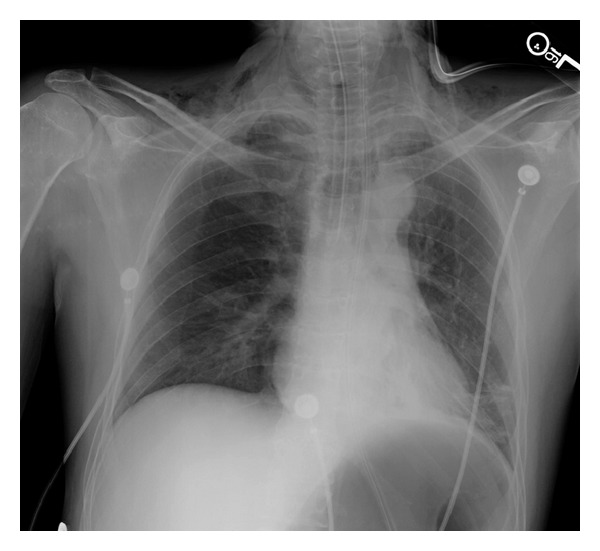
X-ray chest AP view showing endotracheal tube in the right mainstem carina.
